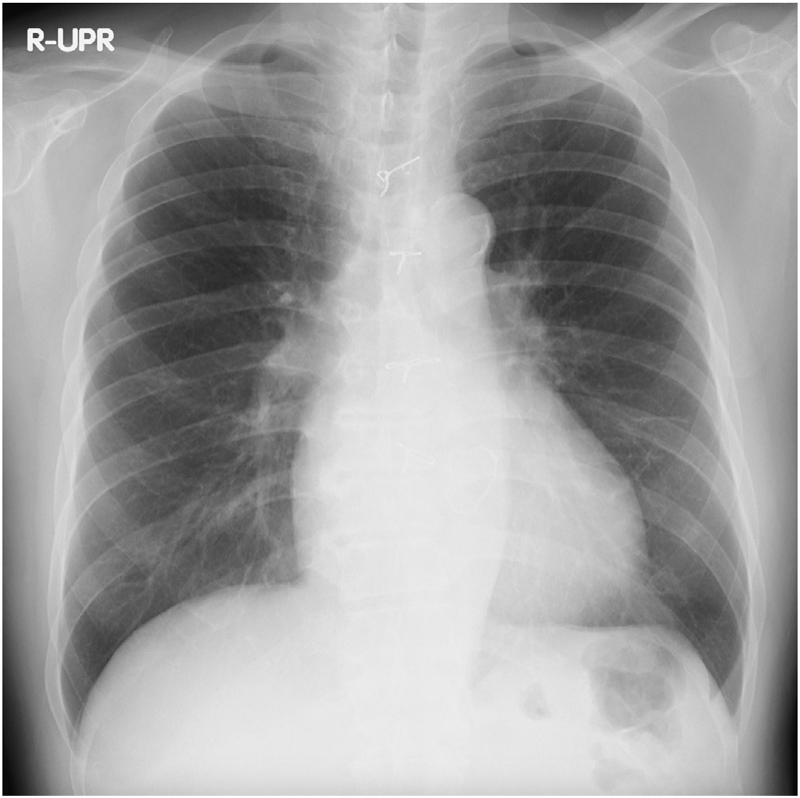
Chest Radiograph on Admission The chest radiograph revealed cardiac enlargement without congestion or pleural effusion.
